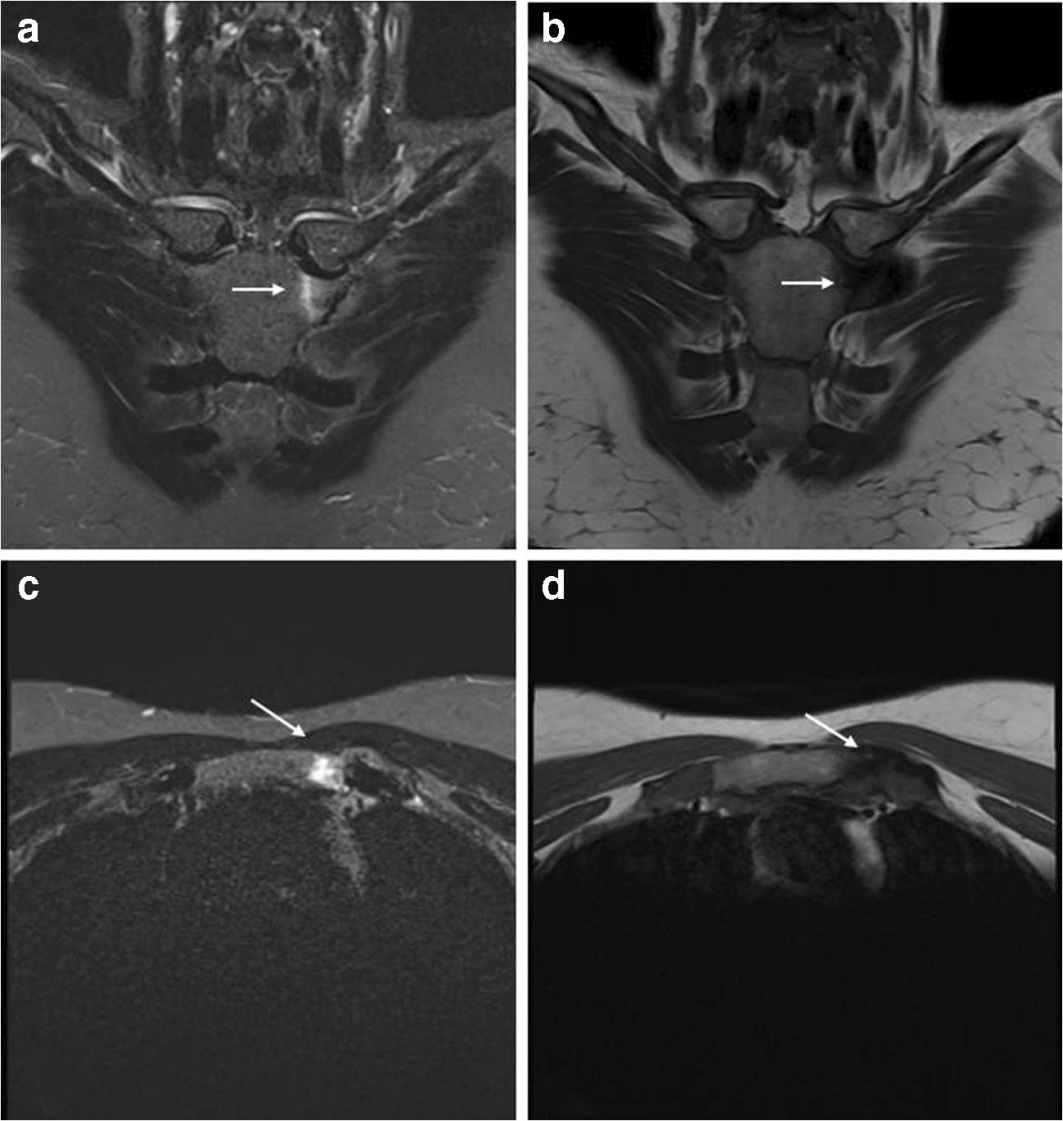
a, b, c, d Inflammatory. A 49-year-old woman with swelling and pain in the left sternoclavicular joint and history of psoriasis. MRI highlights the ELMSI of the joint (*arrows*), which is hyperintense in STIR (a–c) and hypointense in T1w (b–d) imaging. This is a very particular condition and can be referred to either an early SAPHO (synovitis-acne-pustulosis-hyperostosis-osteitis) syndrome, which is often connected with a special form of psoriasis (pustulosis palmoplantaris) and has an inflammatory involvement of the sternoclavicular joint, or a Tietze syndrome, a painful inflammation that can affect the costochondral, costosternal, or sternoclavicular joints
